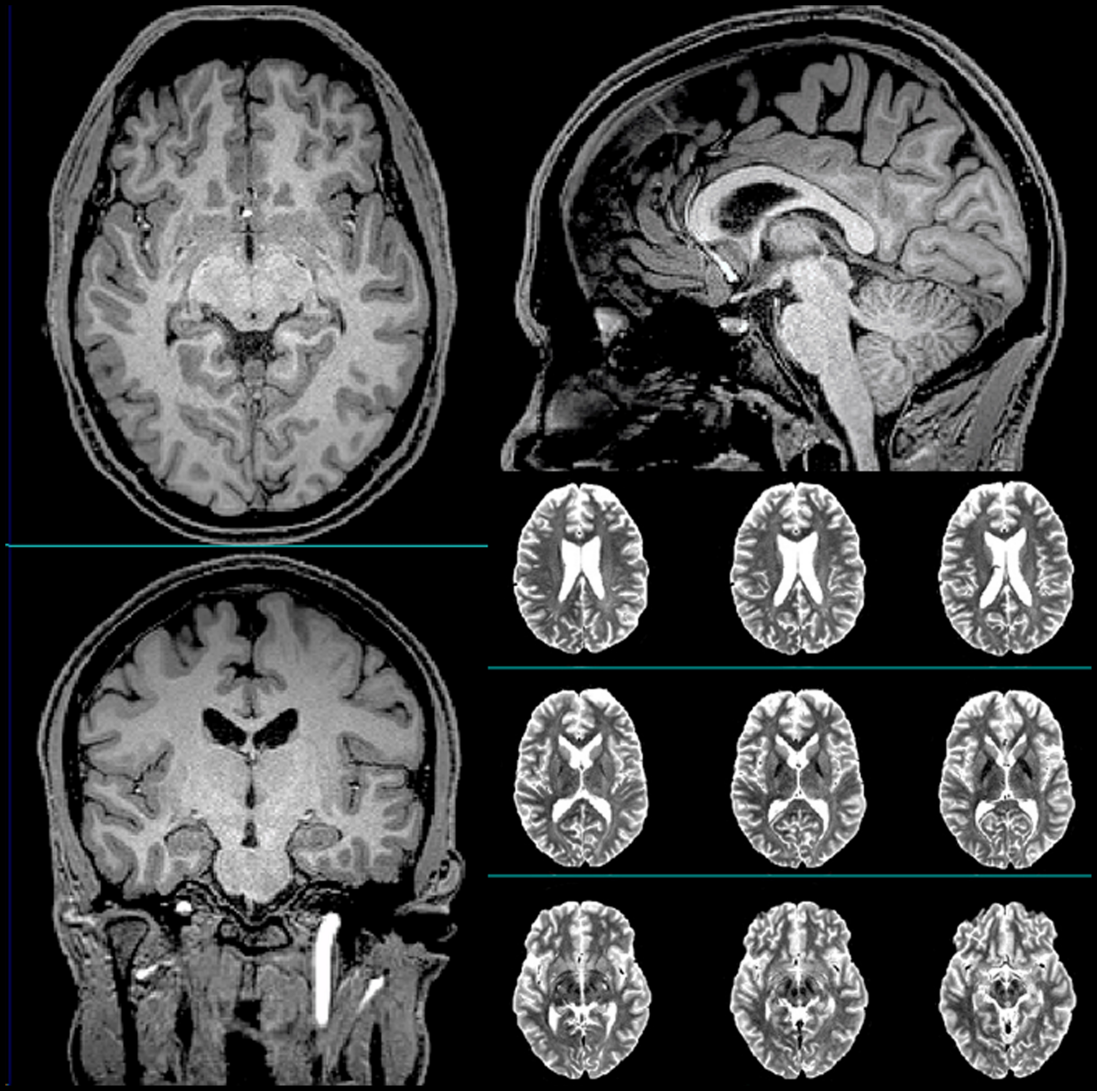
Representative T1-weighted (axial, sagittal, and coronal) and T2-weighted (axial) anatomical scans of the subject acquired at baseline.
